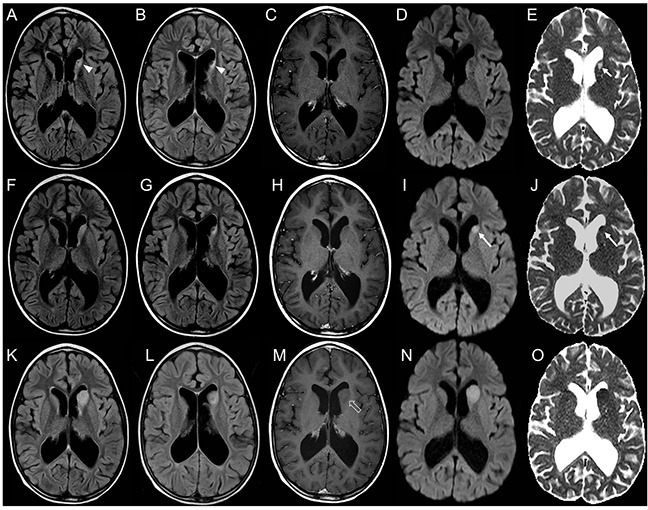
Brain MRI findings in an 8-year-old boy with focal distant medulloblastoma relapse detected earlier by DWI Axial FLAIR (A, B) and post-contrast T1-weighted (C) images reveal a small focal gliotic area in the head of the left caudate nucleus (arrowheads, A, B). DWI (D) and corresponding ADC (E) map show facilitated diffusion (short thin arrow, E). This sequela was stable for 2 years without evidence of disease relapse. Subsequent brain MRI (F-J) does not reveal significant changes when compared to the prior study on both FLAIR (F, G) and post-contrast T1-weighted images (H). At that time conventional pre- and post-contrast MRI were considered negative for relapse. On the contrary DWI (I) and ADC (J) map clearly show a change in signal intensity of the left caudate head nucleus focal lesion, demonstrating decreased diffusivity (long thin arrows, I, J); evaluation of DWI/ADC images was considered positive for relapse. Follow-up MRI (K-O) shows increased volume of the focal lesion, with persistent lack of contrast enhancement (open arrow, M) and decreased diffusivity (N, O), confirming focal relapse.
